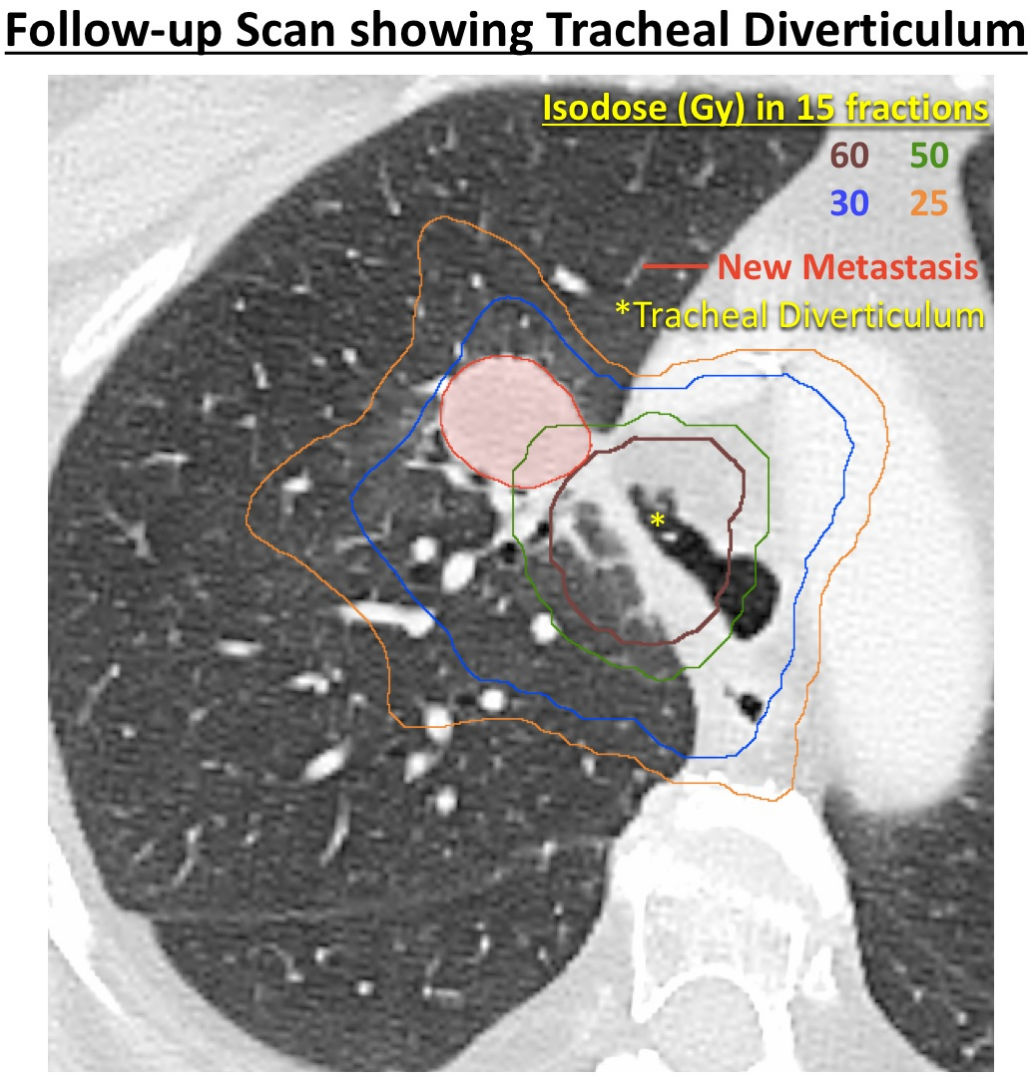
Follow-up CT scan showing tracheal diverticulum in the region of prior high-dose radiotherapy. Prior radiotherapy dose is depicted as isodose levels. A new metastatic nodule is outlined in red.
